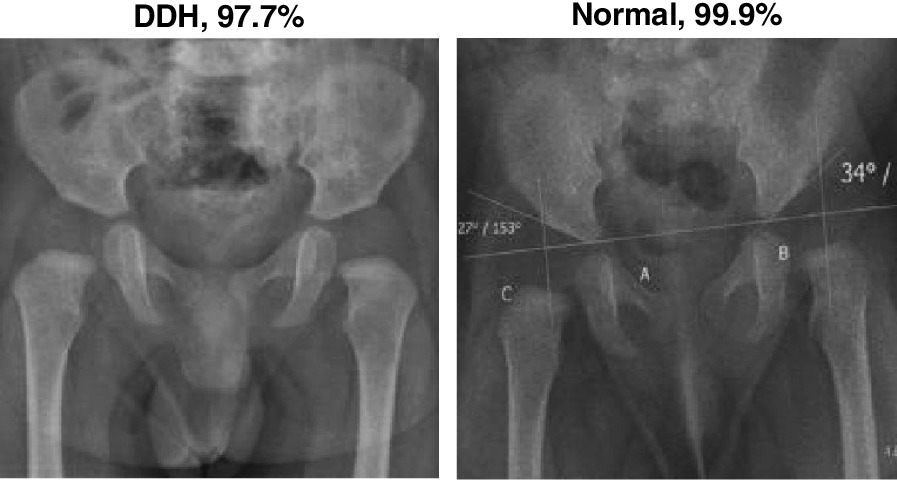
A sample of wrongly classified X-ray images
